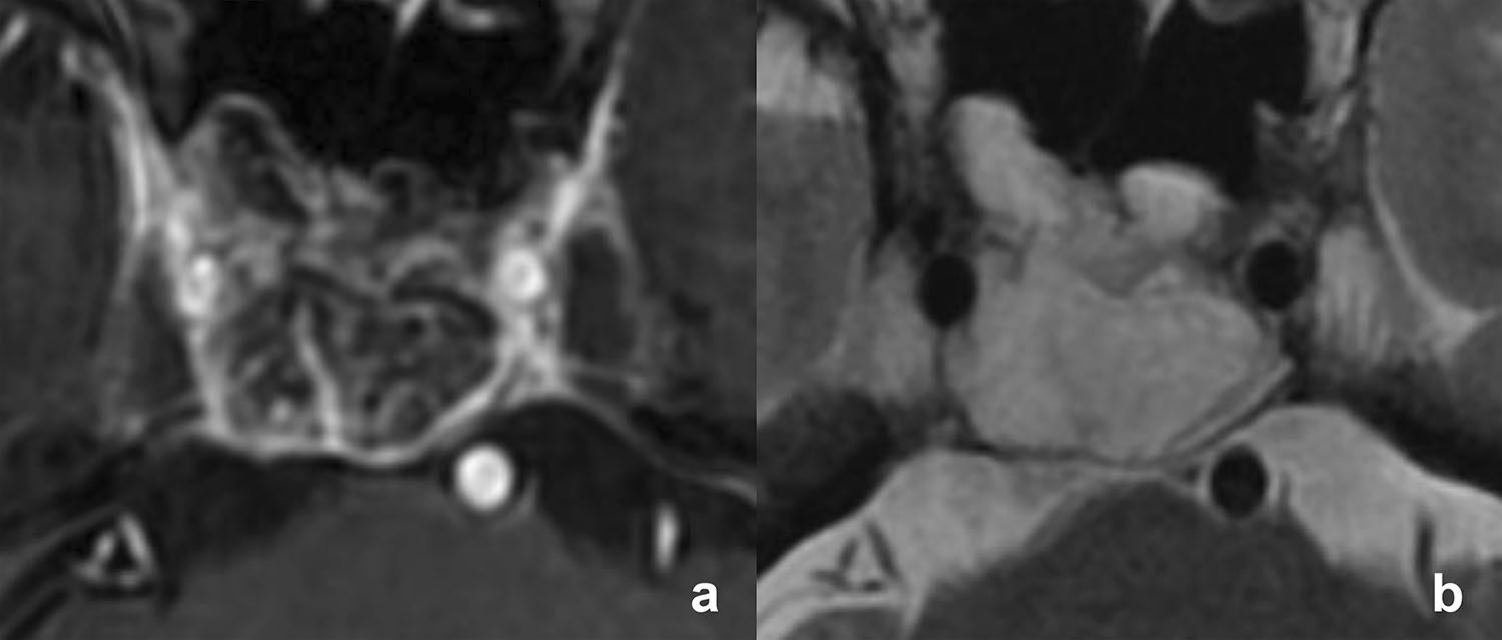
Magnetic resonance imaging of stroma-poor chordoma. (a) Contrast-enhanced T1-weighted and (b) T2-weighted imaging show a poorly enhancing mass originating from the clivus with T2 high signal intensity.
